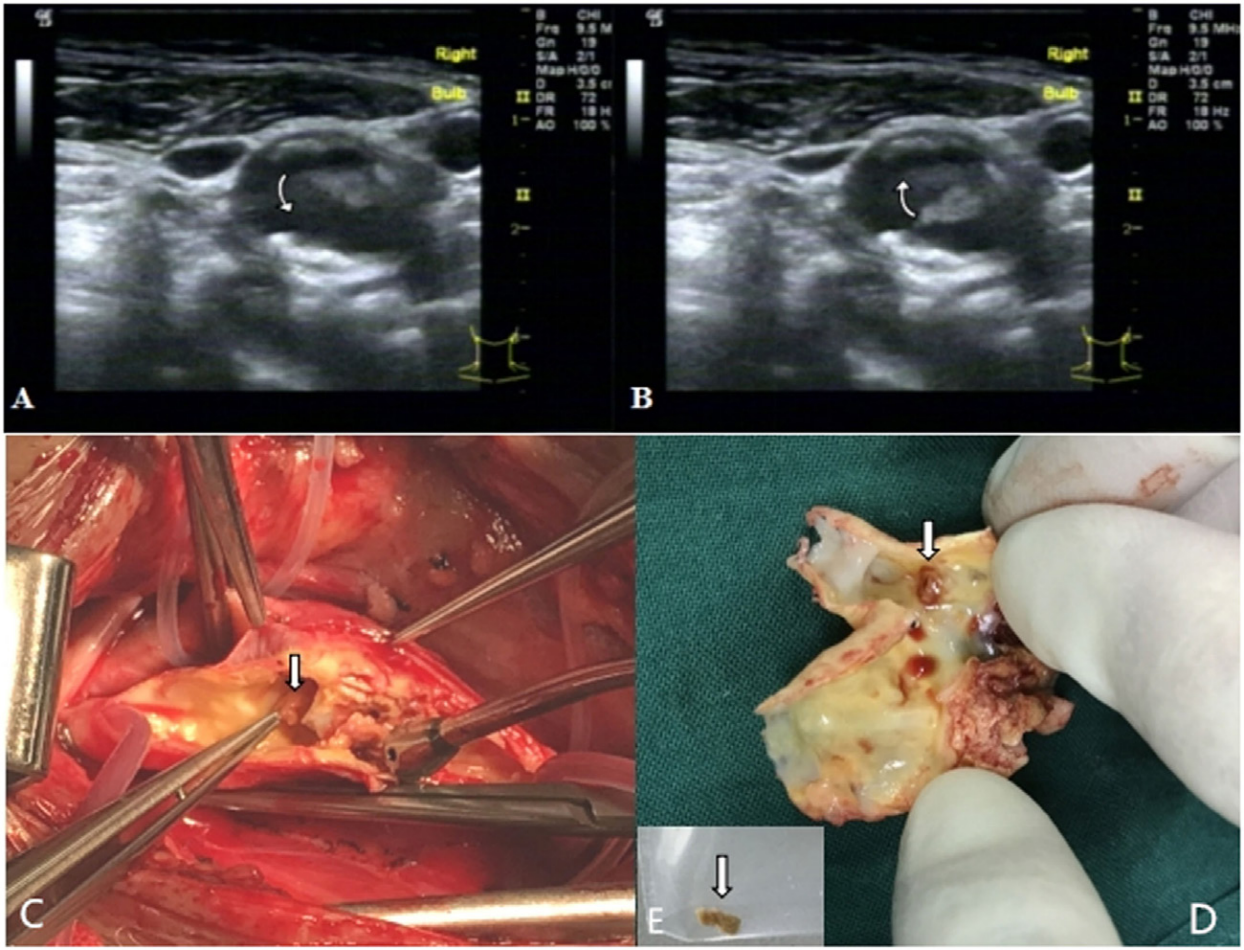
The two-dimensional ultrasonography of right carotid artery shows that the floating carotid plaques in the medial wall of the bifurcation of the distal carotid artery, moving with the cardiac cycle [(A,B) arrows]. The floating material with pedicel can be found in the bifurcation of carotid artery during operation [arrows (C)]. The floating material [arrow (E)] was stripped off from the base of the desquamated plaque [arrow (D)].
